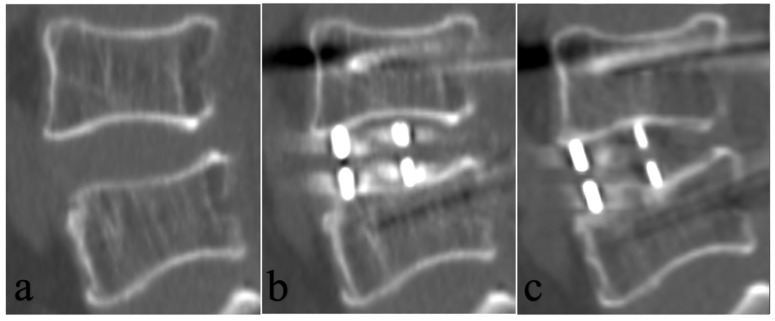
Case 2. Images obtained in a 63-year-old woman with BMD (41.3 mg/cm^3^) and absence of intraoperative endplate injury following OLIF with bilateral posterior pedicle screws fixation. (a) Preoperative CT sagittal reconstruction. (b) CT sagittal reconstruction at 3 days postoperatively. (c) CT sagittal reconstruction at 2.5 years postoperatively showed the presence of CS.
